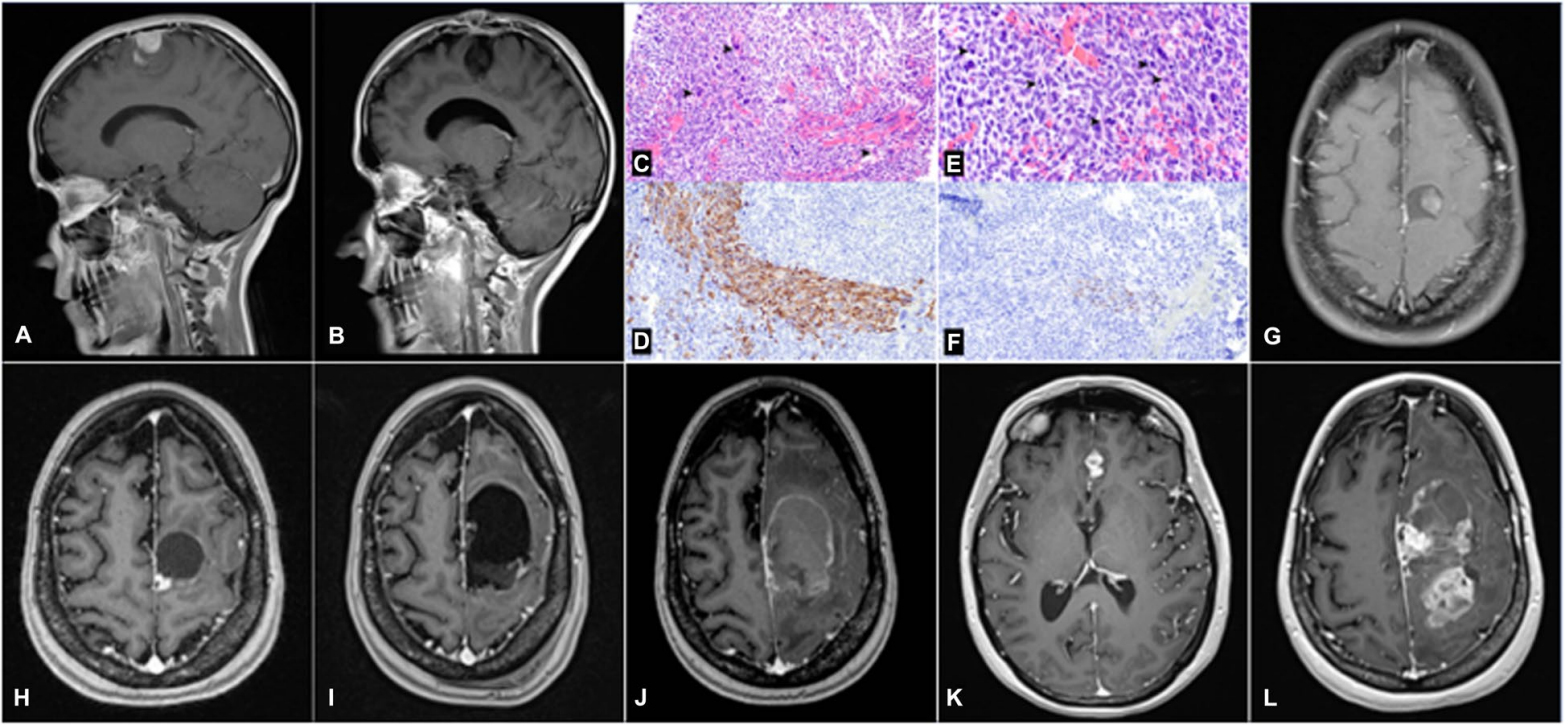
Case 2. Sagittal (a) and axial (b) post-contrast T1-weighted images showing the tumor at the right side extending from T10 to the sacrum. Post-surgical sagittal (c) and axial (d) post-contrast T1-weighted images showing the resection cavity
